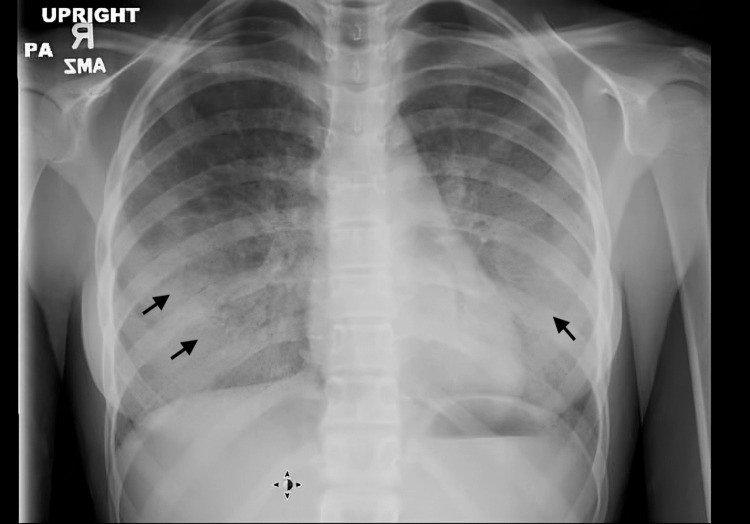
Chest radiograph showing bibasilar infiltrates and interstitial markings
